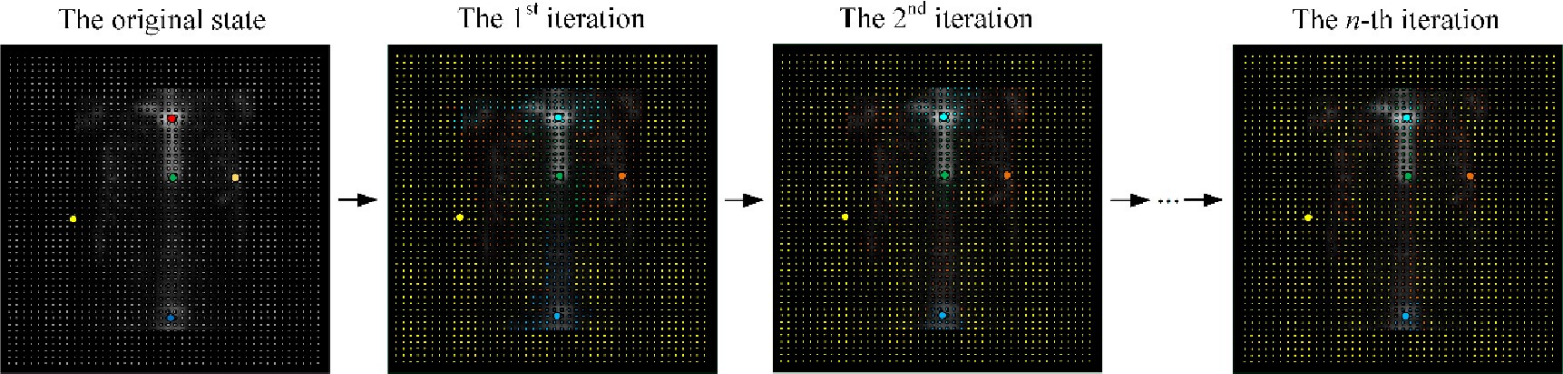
An example of *K*-means clustering based hotspot segmentation with thorax bone SPECT image with *K* = 5.
